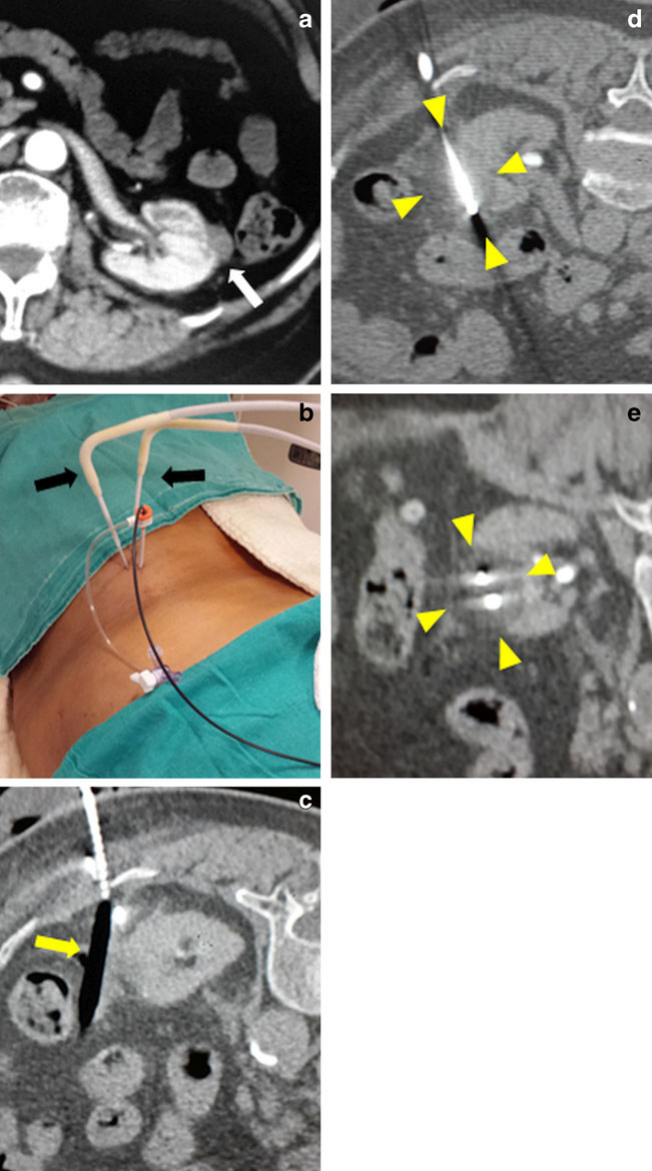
Cryoablation of recurrent renal cancer. a The axial contrast-enhanced CT image shows an exophytic renal tumour (*white arrow*) next to the descending colon. The patient had undergone ipsilateral partial nephrectomy for RCC 6 months earlier. b, c Under local anaesthesia and US+BT guidance, two cryoneedles are inserted percutaneously (*black arrows*) into the lesion. The tumour is separated from the descending colon with an angioplasty balloon (*yellow arrow*) inserted percutaneously through a sheath. d, e Axial and reformatted CT images show the iceball (*arrowheads*) covering the tumour and the two cryoablation needles. During the 1.5-year follow-up, there was no evidence of tumour recurrence
